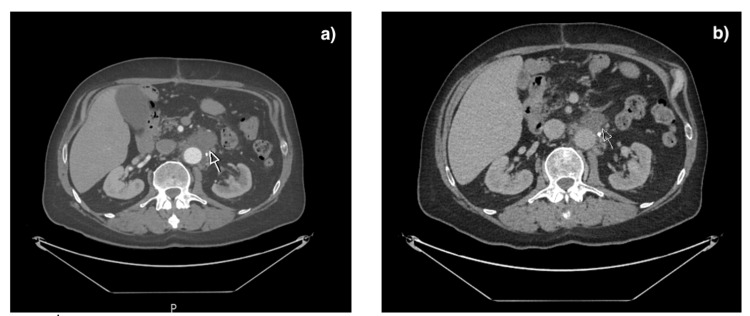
Computed tomography (CT) scan of abdomen and pelvis a: postoperative peri-graft collection (arrow); b: improvement in peri-graft collection three months postoperatively (arrow).
